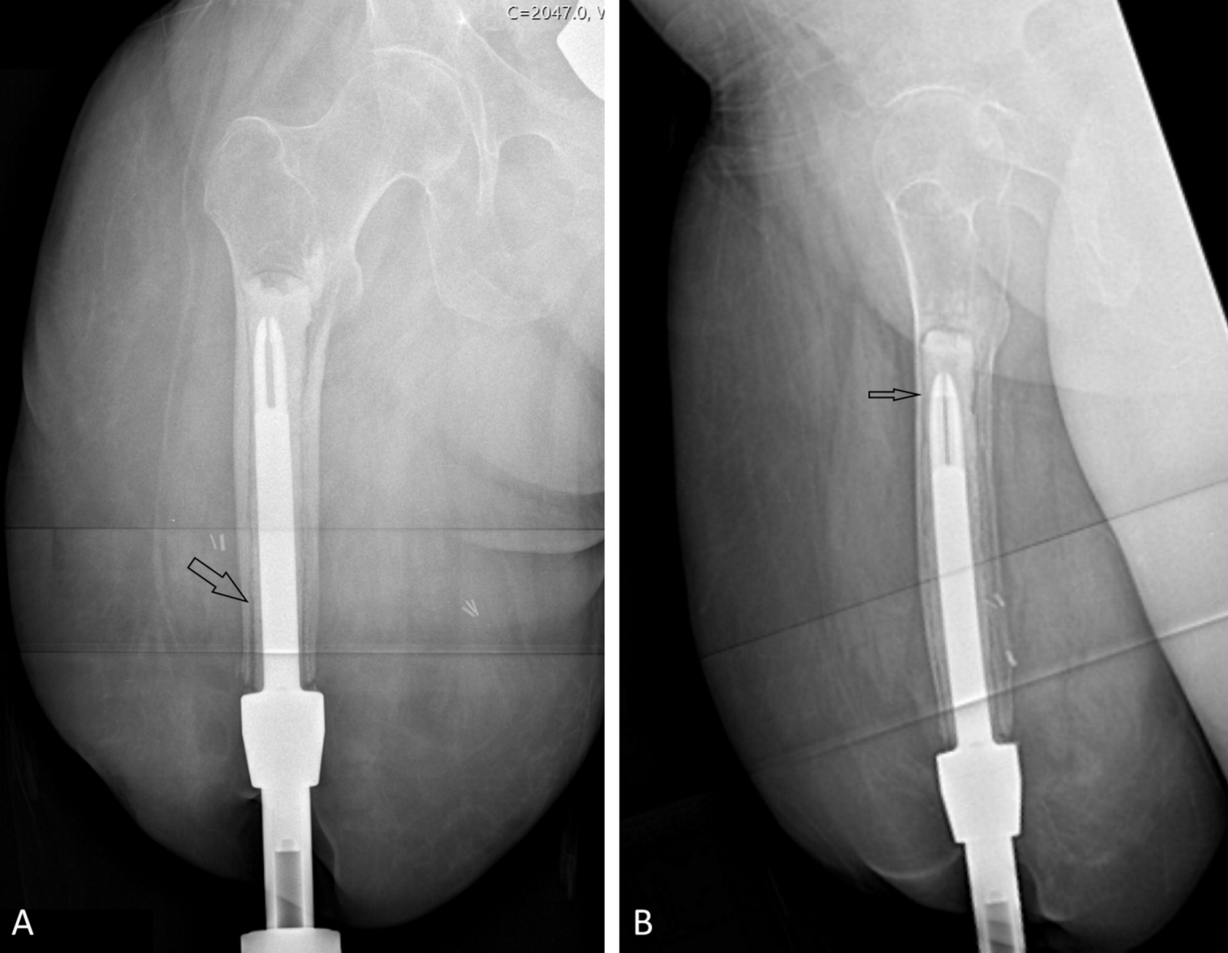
Radiographs of the right femur with a cemented osseointegrated prosthesis at six months. Arrows on the anterior-posterior (A) and lateral (B) views identify a loose cement mantle.
